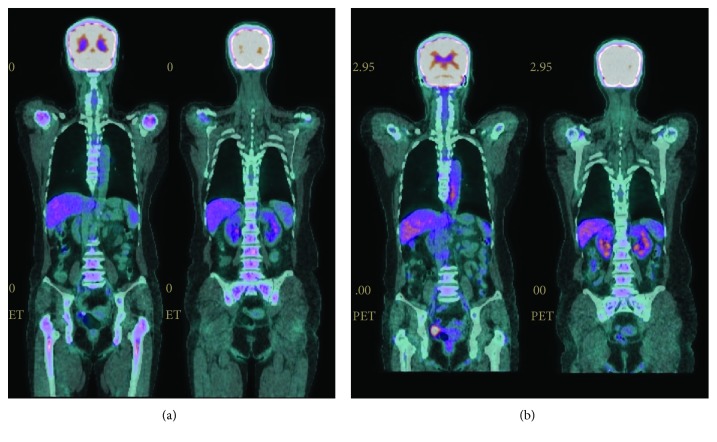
PET/CT scans. (a) Profuse bone marrow uptake (SUVmax: liver 3.1, skeleton 4.2) 15 days after starting treatment with tamoxifen, October 16, 2017. (b) Obvious resolution of previous bone marrow uptake with some residual activity in the lumbar spine (SUVmax: liver 3.3, skeleton 3.2) while on continuous tamoxifen treatment, March 5, 2018.
